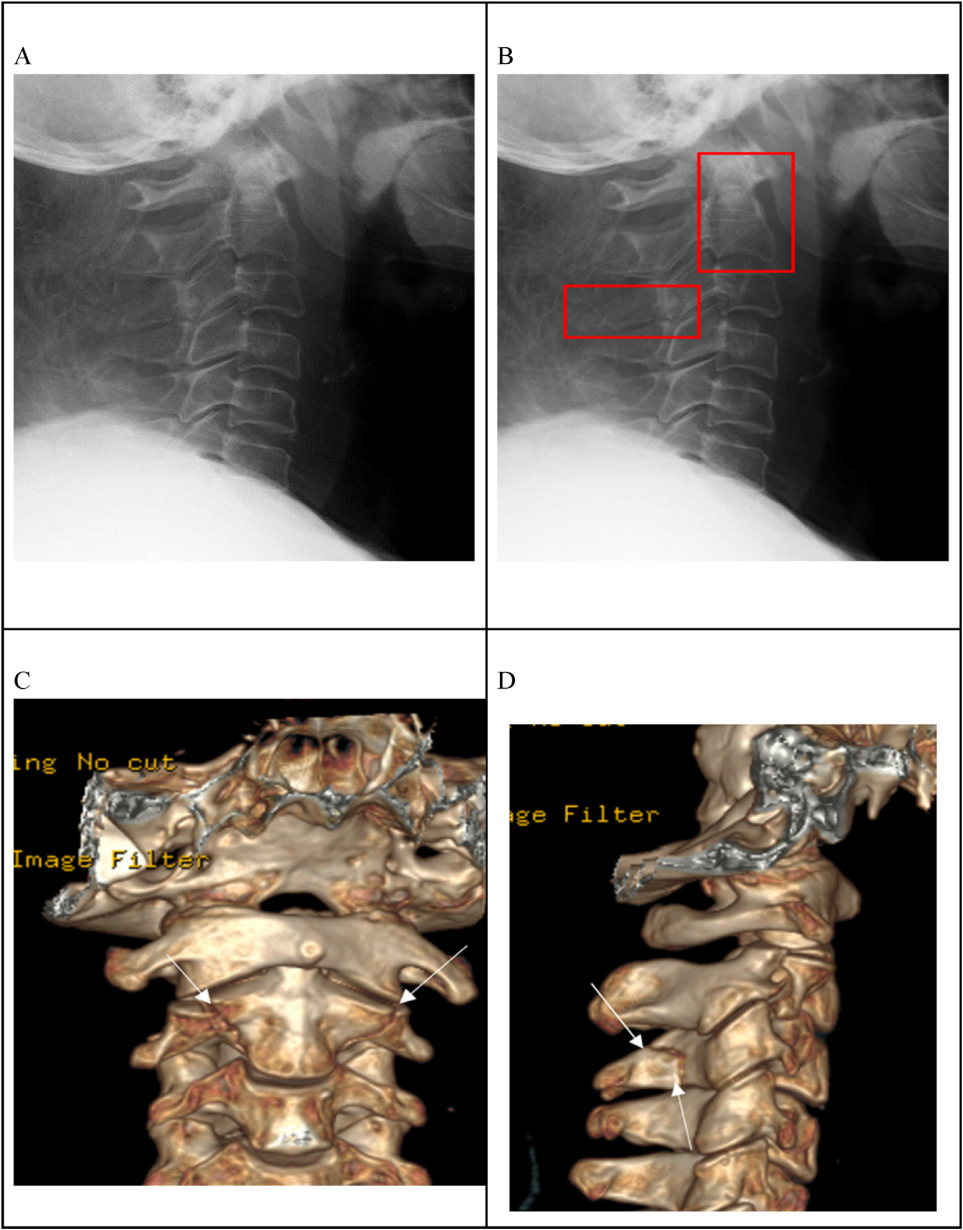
Lateral cervical radiograph with a CT report of fracture at C2 vertebral body and fracture of C3 transverse process. The fractures are not clearly visible on the radiograph (A) and were labeled at the relevant anatomy(B). (C) and (D) 3D CT scan of this patient shows fractures at C2 vertebral body and C3 spinous process.
